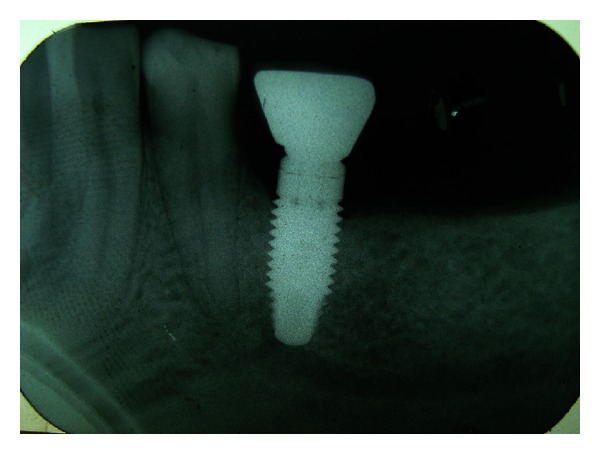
IOPA radiograph 3 months after prosthetic rehabilitation.
